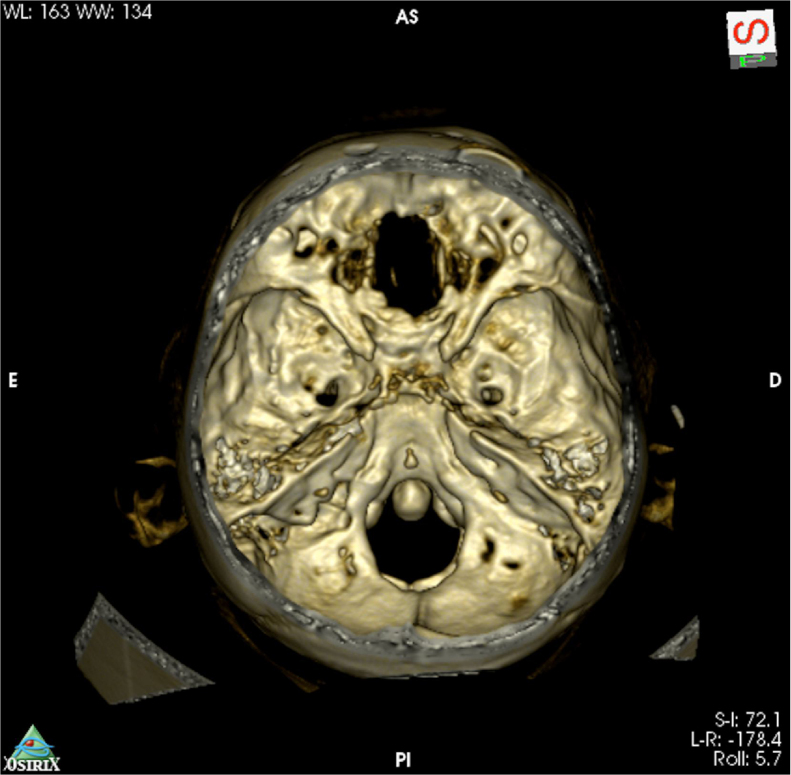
Tridimensional reconstruction of postoperative high definition head CT with a large bone defect from the planum sphenoidale to the crista galli.
